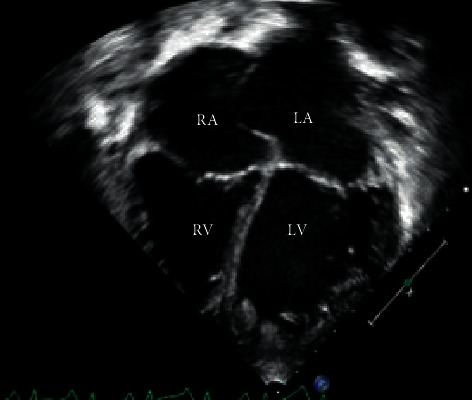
Apical four chamber view showing bilateral atrial and ventricular dilation with anterior bowing of the interatrial septum suggestive of elevated left atrial pressure. There are also prominent hypertrabeculations at the apex of the left ventricle. LA: left atrium. LV: left ventricle. RA: right atrium. RV: right ventricle.
